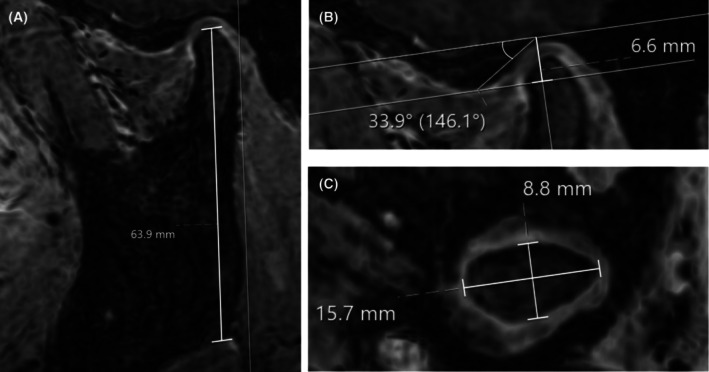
Measurements of osseous temporomandibular joint structures on magnetic resonance images. (A) Ramus height measured on sagittal‐oblique minimum intensity projection (minIP) image. (B) Glenoid fossa depth and articular eminence inclination angle measured on sagittal‐oblique multiplanar reconstruction (MPR) image. (C) Condylar width and depth of the mandibular condyle measured on axial MPR image from a 3D gradient echo sequence.
